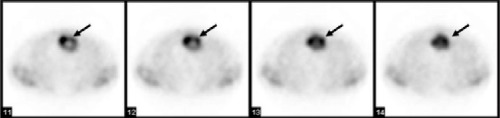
Tc-99m MIBI transaxial images show abnormal increasedactivity located in the anterior mediastinum corresponding to themass revealed on CT in Figure 2
